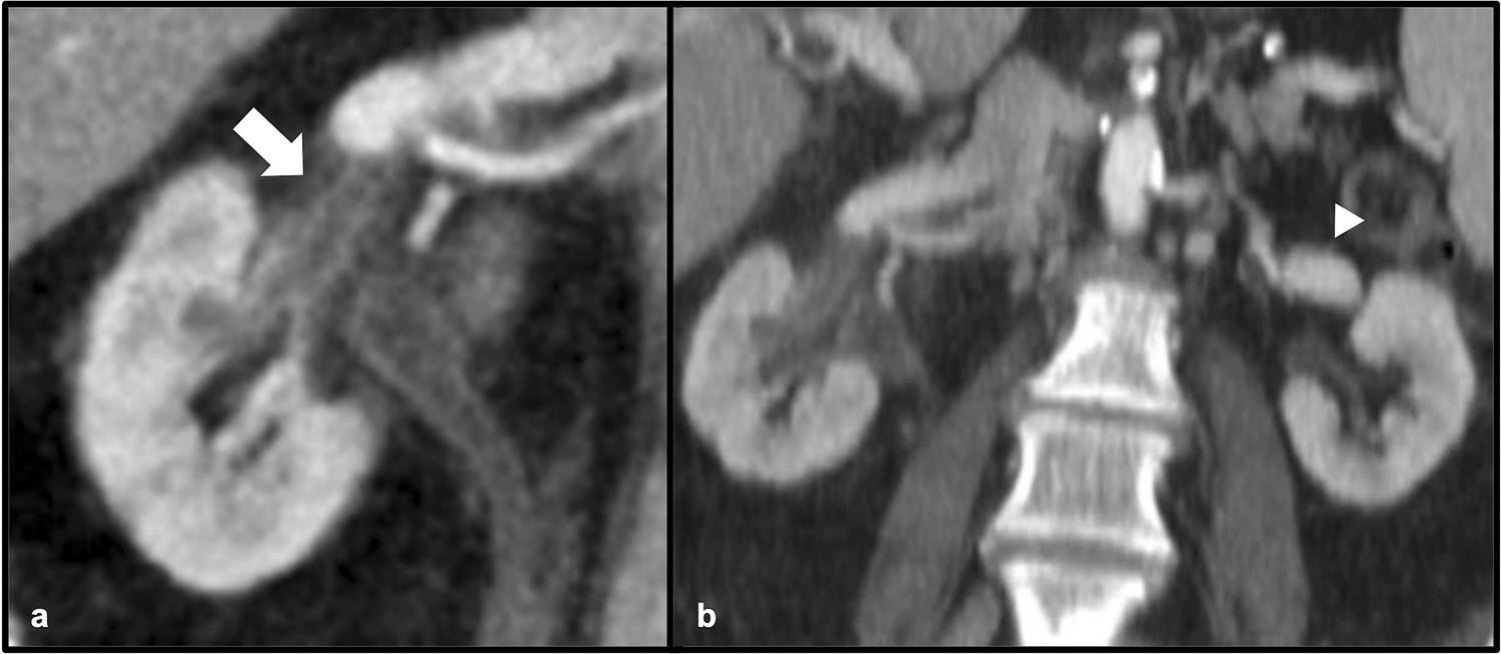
80-year-old male with a remote history of pulmonary squamous cell carcinoma status-post recent resection of benign pulmonary nodule who presented with fever, found to have methicillin-sensitive *Staphylococcus aureus* bacteremia. Coronal post-contrast CT (a) demonstrates a filling defect (white arrow) within a tributary of the right renal vein, with adjacent fat stranding, consistent with septic thrombophlebitis. Coronal post-contrast CT (b) demonstrates the extent of the right renal vein thrombosis. Note the normal-appearing left renal vein (white arrowhead) for comparison
